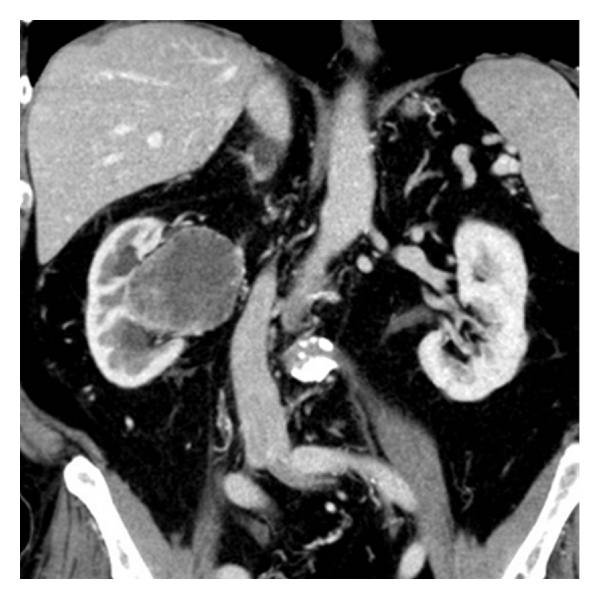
Contrast-enhanced CT abdomen and pelvis showing a tumour with central necrosis originating from the right renal pelvis and protruding into perirenal fat medially. There is slight dilatation of the superior and inferior calyces.
